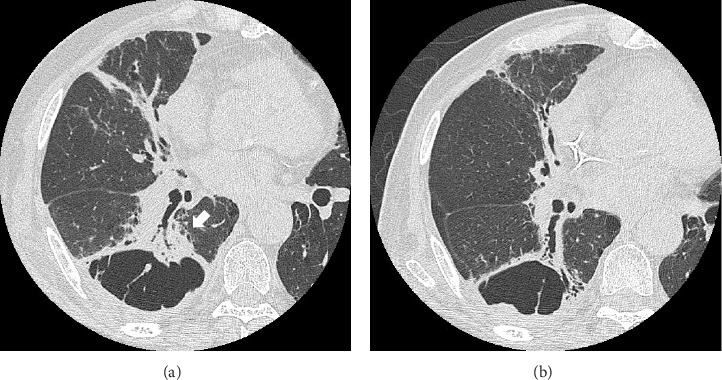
High-resolution computed tomography (HRCT) reveals (a) a thick-walled cystic space with an exophytic enlarged solid nodule (arrow) along the cyst wall and a new shadow of pseudotumor mimicking consolidation in the right lower lobe during third-line chemotherapy; (b) HRCT showing the diminished nodule adjacent to the thickened cystic airspace wall and the cysts fusing into bizarre shapes after 4 months of starting fourth-line therapy.
